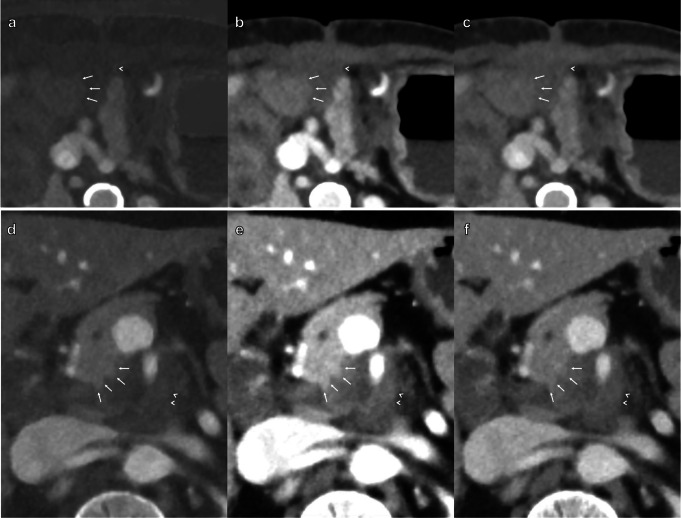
Axial contrast-enhanced abdominal CT scans in portal venous phase. The first column of images (a and d) is MD-iodine, the second column (b and e) VMI at 55 keV, and the third column (c and f) VMI at 70 keV. The first row of images (a-c) is of an 82-year-old female patient with a tumor recurrence post Whipple procedure at the level of the pancreaticojejunostomy. The white arrows indicate the border between the tumor and the jejunal wall. The arrowhead indicates the border between the tumor and the mesenteric fat. The second row of images (d-f) is of a 45-year-old male with a PDAC in the uncinate process of the pancreas encasing the superior mesenteric artery. The white arrows indicate the border between the tumor and normal pancreatic parenchyma. The arrowheads indicate the border between the tumor and the retroperitoneal fat. One of the most experienced readers (Reader 1) graded MD-iodine in the case of the first row as Score 1 for the parameter lesion conspicuity (Lesion not visualized at all), 70 keV as Score 3, and 55 keV as Score 5
